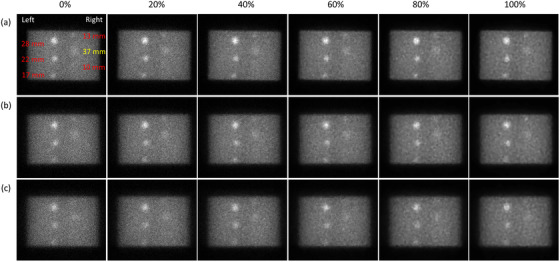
Posterior view of Tc‐99m planar scintigraphy (window level = 50/window width = 100) acquired with a blend ratio of 0, 20, 40, 60, 80, and 100% (from left to right) for (a) NEMA_small_, (b) NEMA_medium_, and (c) NEMA_large_
